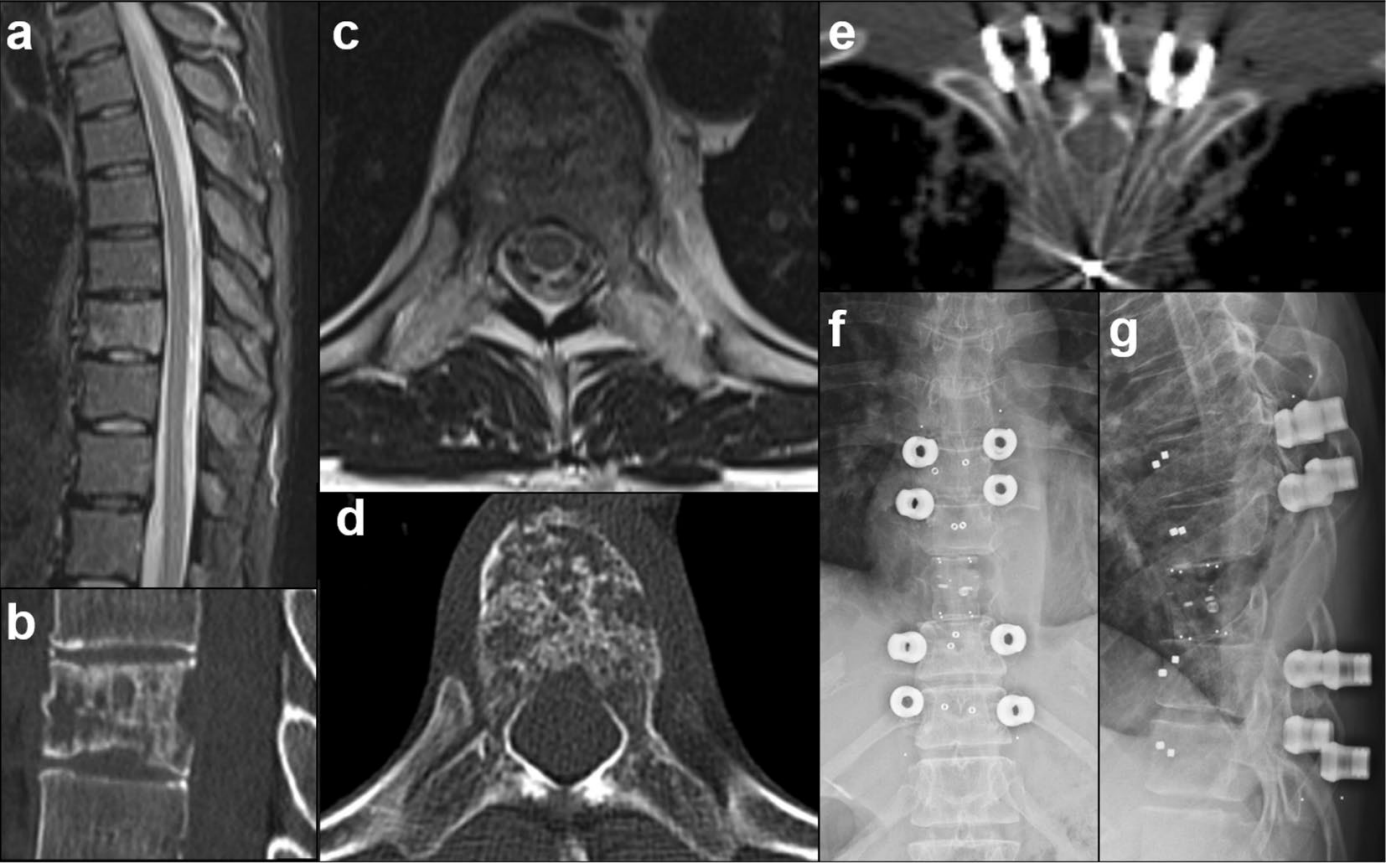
Case example of a 48-year-old female suffering from metastatic breast cancer with a singular metastasis at Th8 (SINS 7, preop. images a–d). The patient suffered from isolated thoracic back pain without neurological deficits and a KPS of 90%. She was treated by en-bloc spondylectomy of Th8 with CFRP vertebral body replacement and iCT-based navigated posterior instrumentation from Th6 to Th10 using CFRP pedicle screws (intraop. imaging e and postop. X-ray f + g). Adjuvant stereotactic radiosurgery was performed using CyberKnife.
